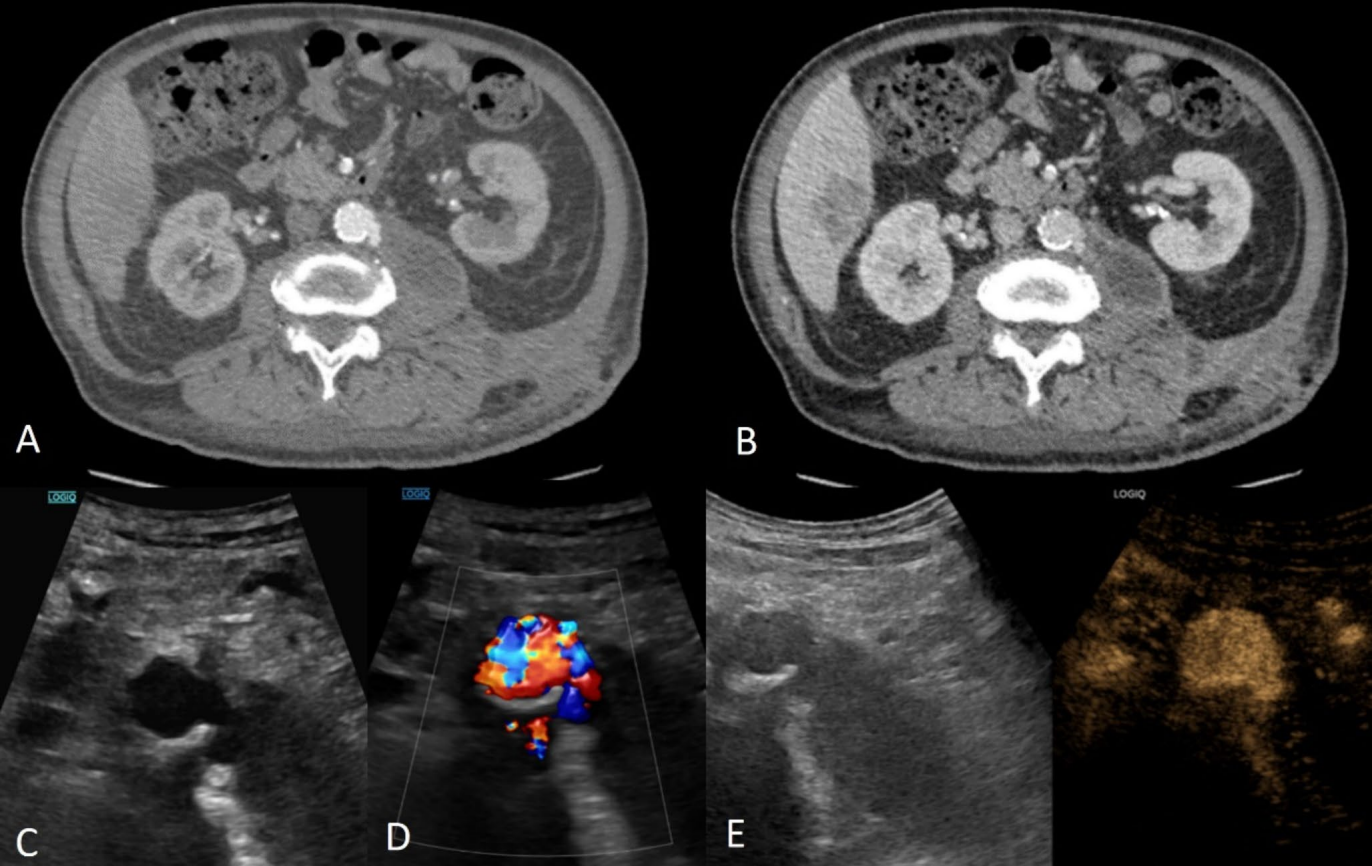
68 year-old male with penetrating atherosclerotic ulcer in the abdominal aorta. Arterial (A) and venous phase (B) CT images showing a focal outpouching of the contrast agent at the level of the infrarenal abdominal aorta. Note the abutting collection within the psoas muscle, probably related to subacute hemorrhagic leaking. There is no pooling of the contrast agent on the venous phase image and therefore constellation of findings are consistent with a penetrating atherosclerotic ulcer. This aortic pathology was followed-up with US including CEUS. B-mode (demonstrating a predominantly hypoechoic lesion causing discontinuity of the aortic wall (A). Color Doppler technique revealed flow reversal inside the penetrating aortic ulcer. CEUS demonstrates enhancement of the lesion with contrast microbubbles but confidently excludes contrast extravasation to suggest active hemorrhage at the time of the exam since there is no contrast opacification appreciated outside of the penetrating ulcer contours or within the abutting psoas collection
